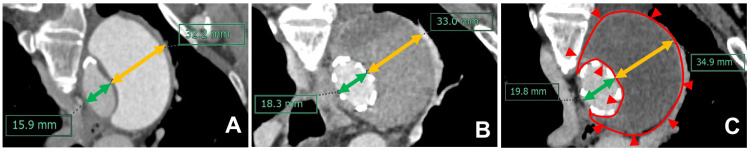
Serial contrast-enhanced computed tomography (CT) images of case 1. A: Preoperative CT. The true lumen diameter was 15.9 mm (green line), while that of the false lumen was 32.2 mm (yellow line). B: CT before the patient’s discharge from the hospital. The true lumen diameter increased slightly to 18.3 mm (green line), while that of the false lumen increased to 33.0 mm (yellow line). Although no reduction in false lumen size was observed, favorable thrombosis was achieved. C: CT performed at six months postoperatively. The true lumen diameter had further increased to 19.8 mm (green line), while that of the false lumen measured 34.9 mm (yellow line). The false lumen remained well thrombosed (the red outlined area), with continued expansion of the true lumen.
